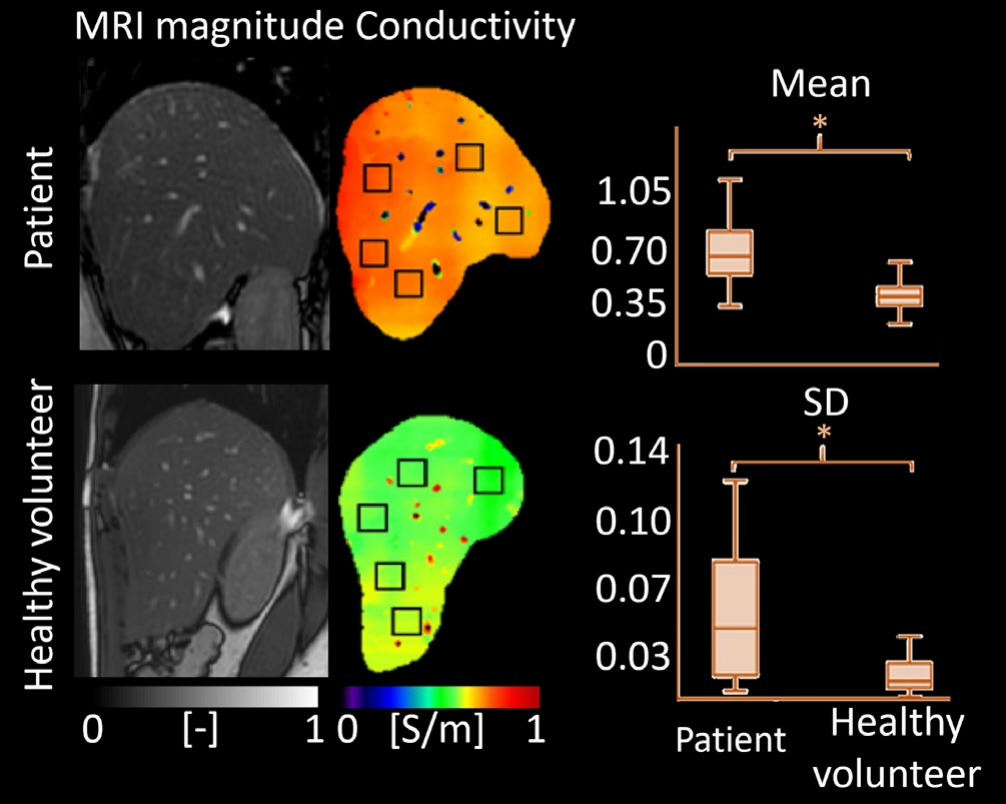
MRI bFFE magnitude images and conductivity maps of a healthy volunteer and a patient with hepatic cirrhosis (Stage F4). Conductivity of the liver parenchyma of the patient is much higher than that of the volunteer and shows much more variability (standard deviation—SD) in selected regions of interest (black squares), p* < 0.001 (adapted from [64]).
